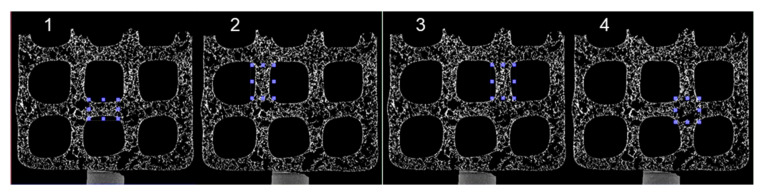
Parts of samples chosen for 3D pore morphology analysis by X-ray micro tomography (marked with blue in images 1–4).
